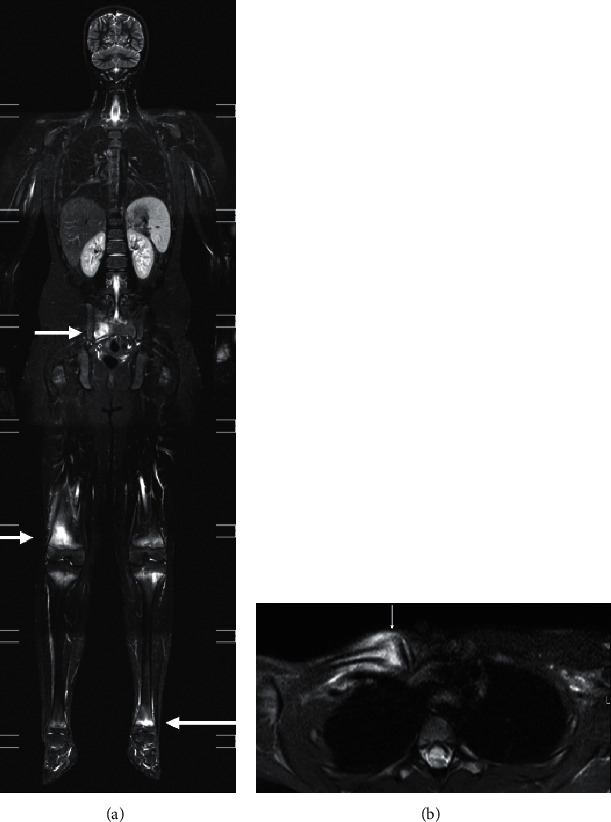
CRMO. Coronal whole-body MRI of an 8-year-old boy (a) with CRMO initially presenting with right knee pain. Note the multifocal involvement which included both knees, left ankle, and the sacrum (arrow). Axial STIR (b) of a different CRMO patient aged 7, with classic involvement of right medial clavicle with associated hyperostosis (arrow).
